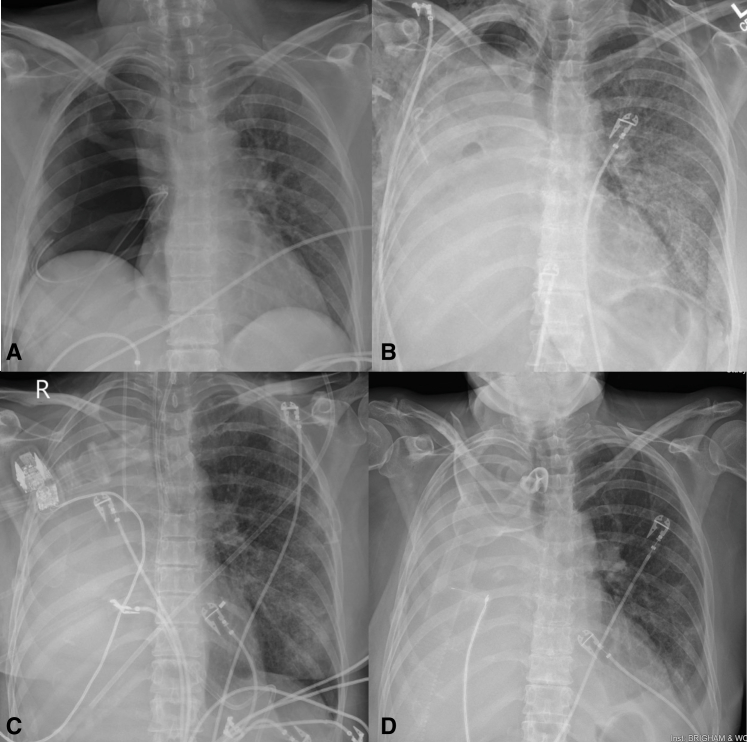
Postpneumonectomy radiograph evolution. A, POD 0 Right pneumonectomy. B, POD 12 single-lung ARDS. C, POD 18 before ECCO_2_R with refractory hypercapnic respiratory failure despite maximal ventilatory support. D, POD 30 after ECCO_2_R improving to tracheostomy collar. *POD*, Postoperative day; *ARDS*, acute respiratory distress syndrome; *ECCO2R*, extracorporeal carbon dioxide removal.
